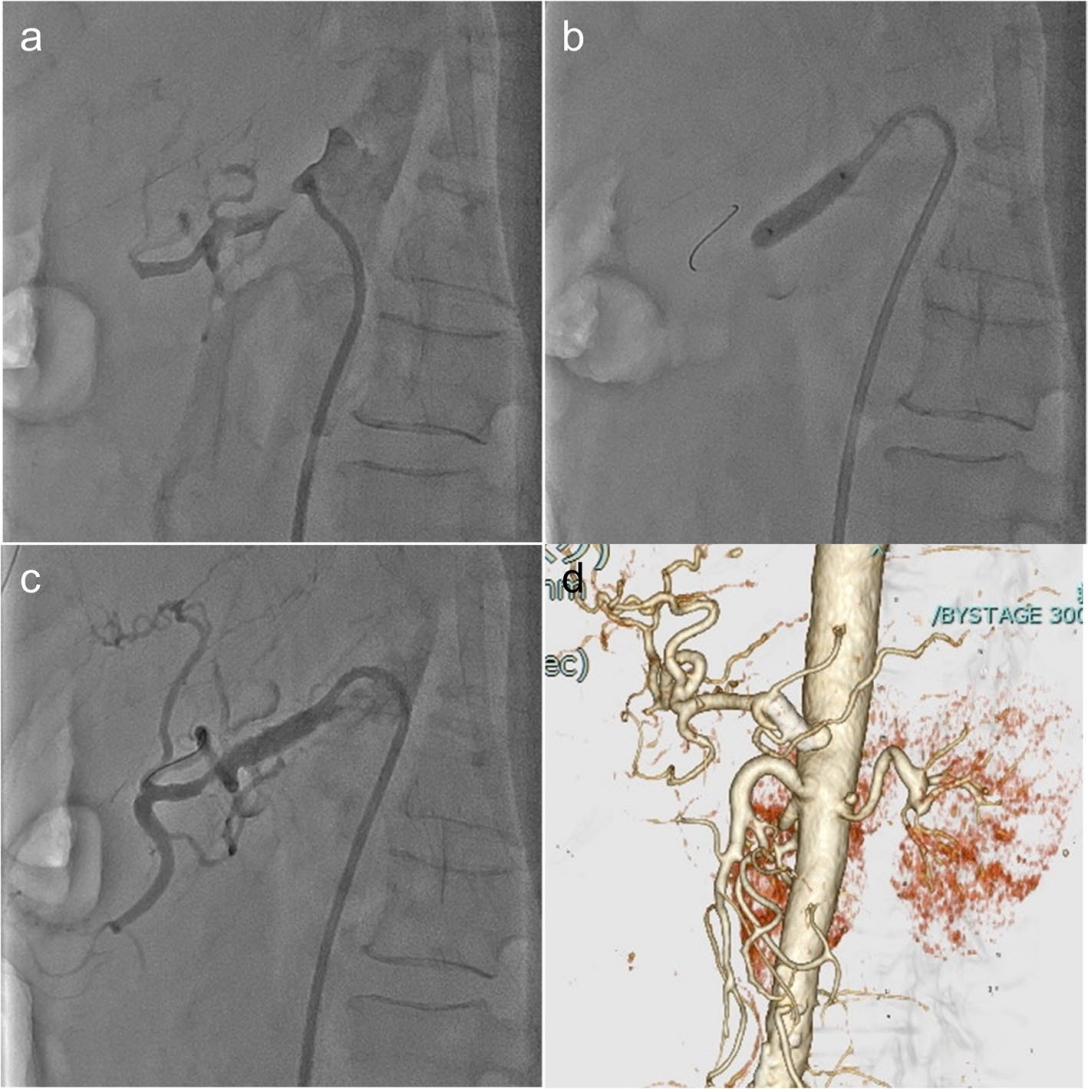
a, b, c, and d Second endovascular treatment and final CT findings. a The re-occluded celiac artery. b A long balloon expandable stent was deployed. c Improved blood flow after stenting. d CT findings after stenting
